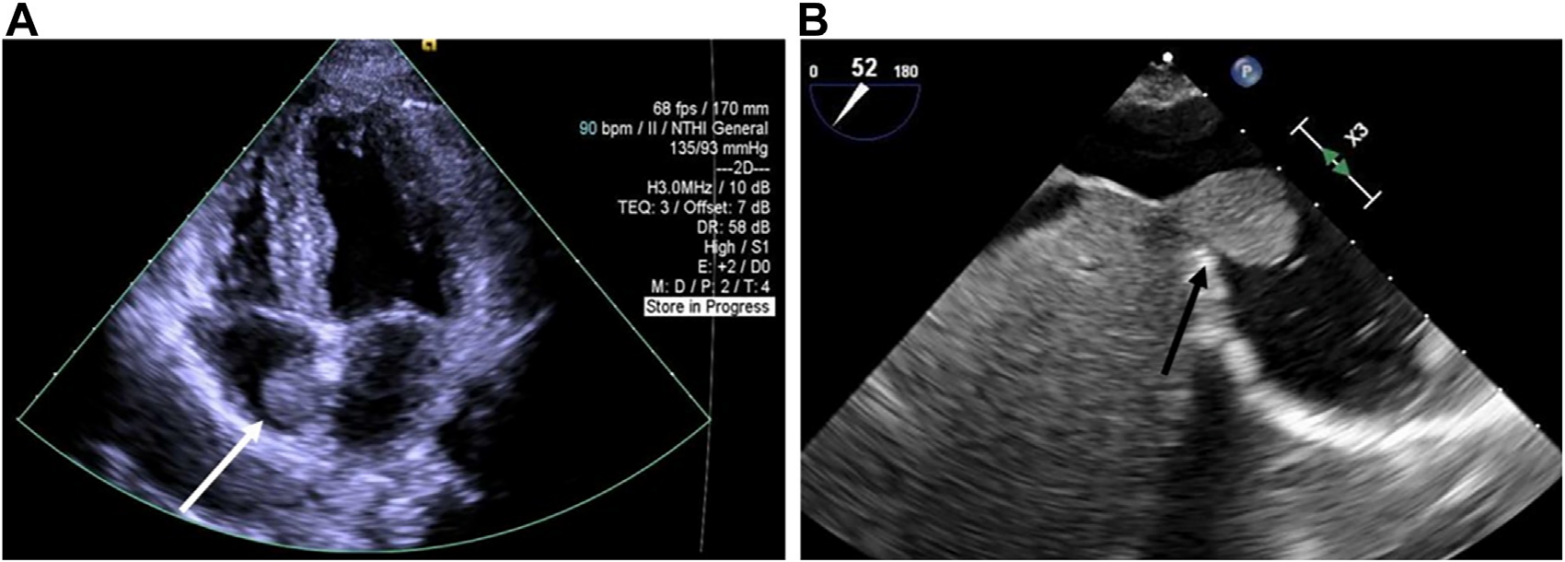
(A) Mobile mass in the right atrium measuring 2.7 × 2.2 cm on transthoracic echocardiogram (arrow). (B) On intraoperative transesophageal echocardiogram, the mass was better visualized close to the liver (arrow).
